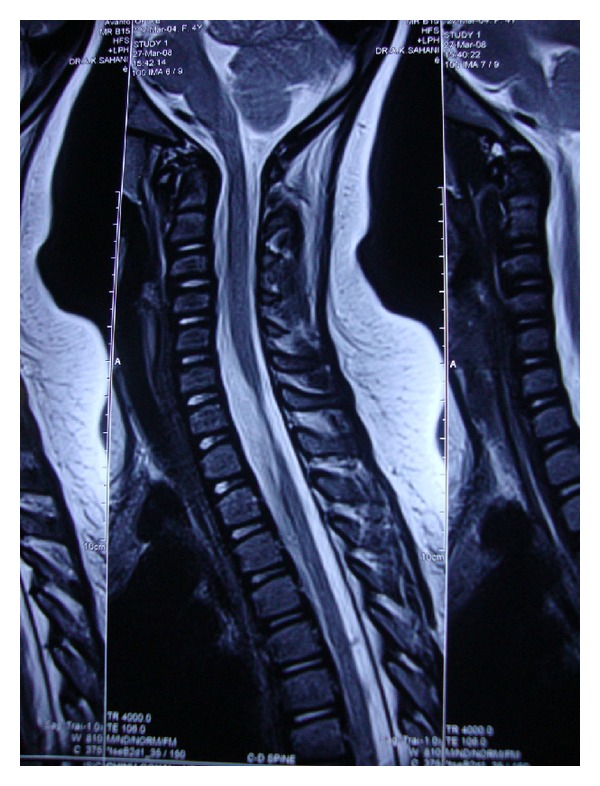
MRI of spine showing injury from C7 to D1.
